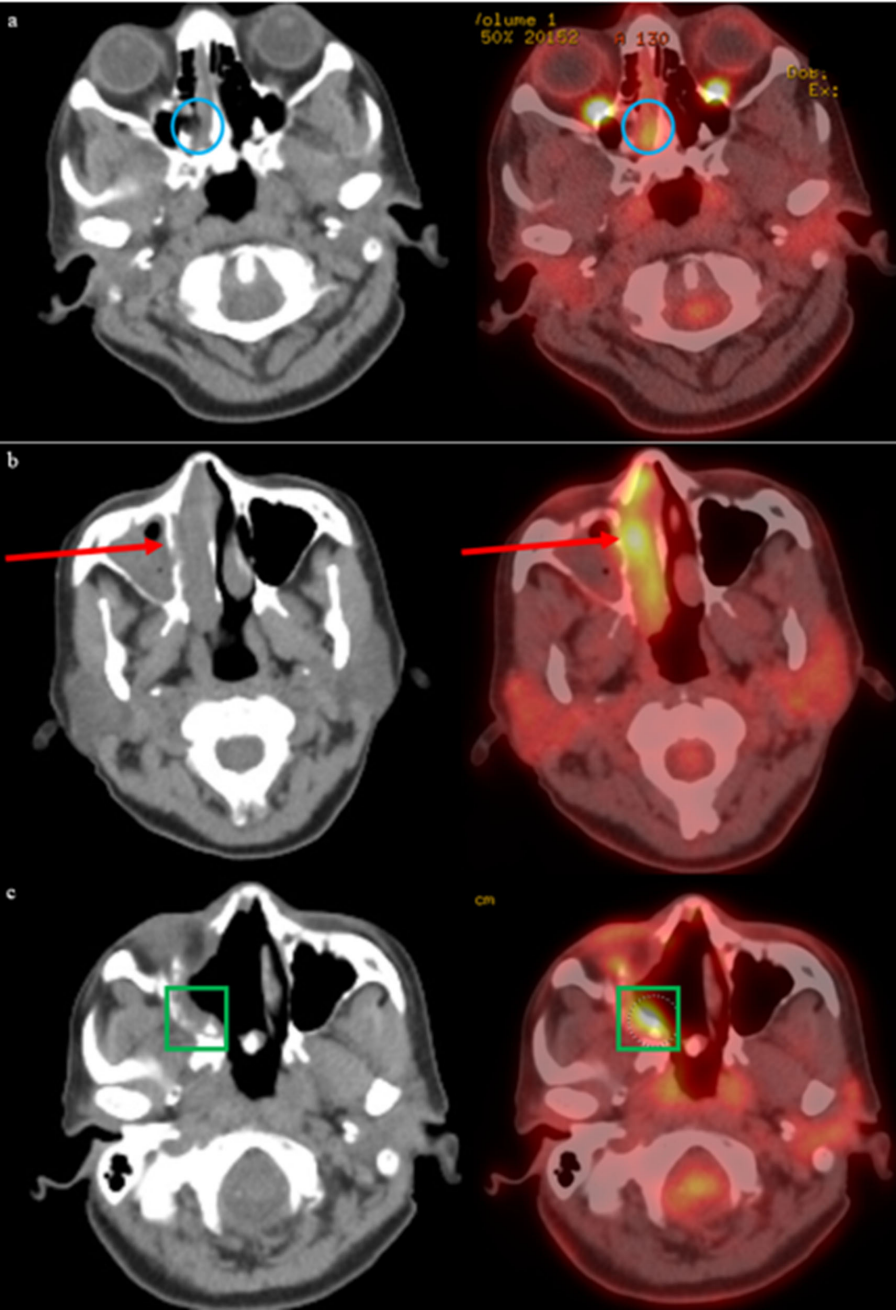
Progression of biopsy-confirmed melanoma in right nasal cavity is detected by initial imaging findings revealing T3 disease. Post-surgical changes in sinus and surrounding soft tissues is observed with PET-CT, with concern for residual disease. (a) Initial PET-CT on 8/26/2021 reveals a foci of asymmetric metabolism corresponding to the soft tissue abnormality in the right nasal cavity (blue circle). (b) Follow-up PET-CT 10/21/2021 revealed increasing soft tissue within the right nasal cavity with heterogeneous activity (red arrow). (c) Postoperative changes related to endoscopic nasal and skull base resection with associated post radiation changes seen on PET-CT dated 1/26/2022, with increased FDG-activity along the right posterior maxillary sinus and right ethmoidectomy bed (green square).
